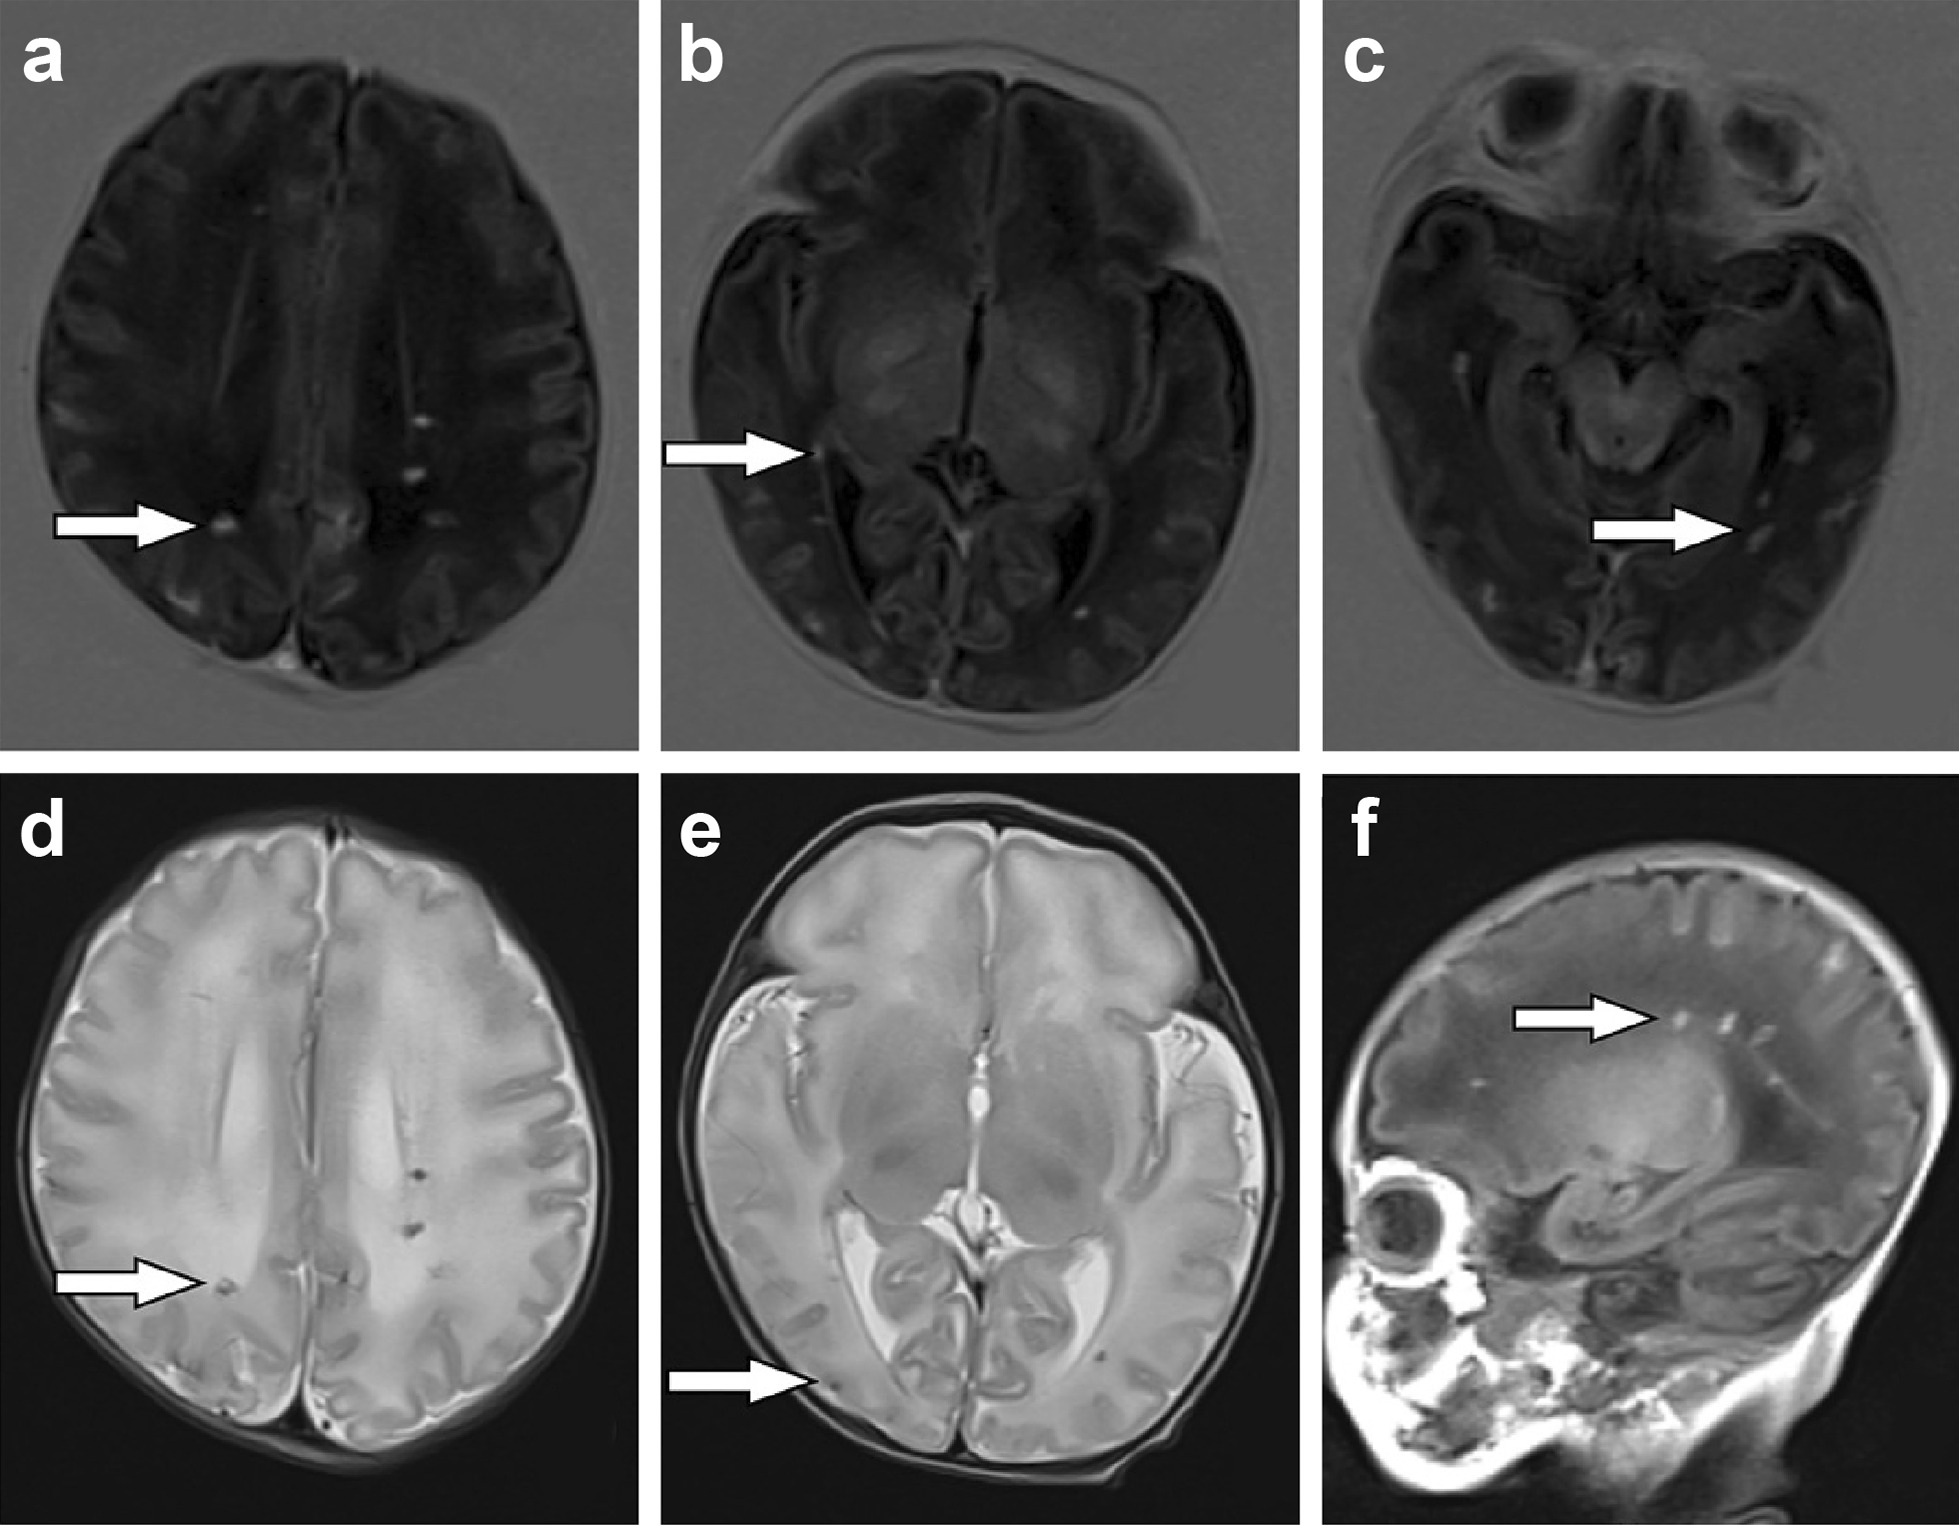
First cranial MRI of the infant
